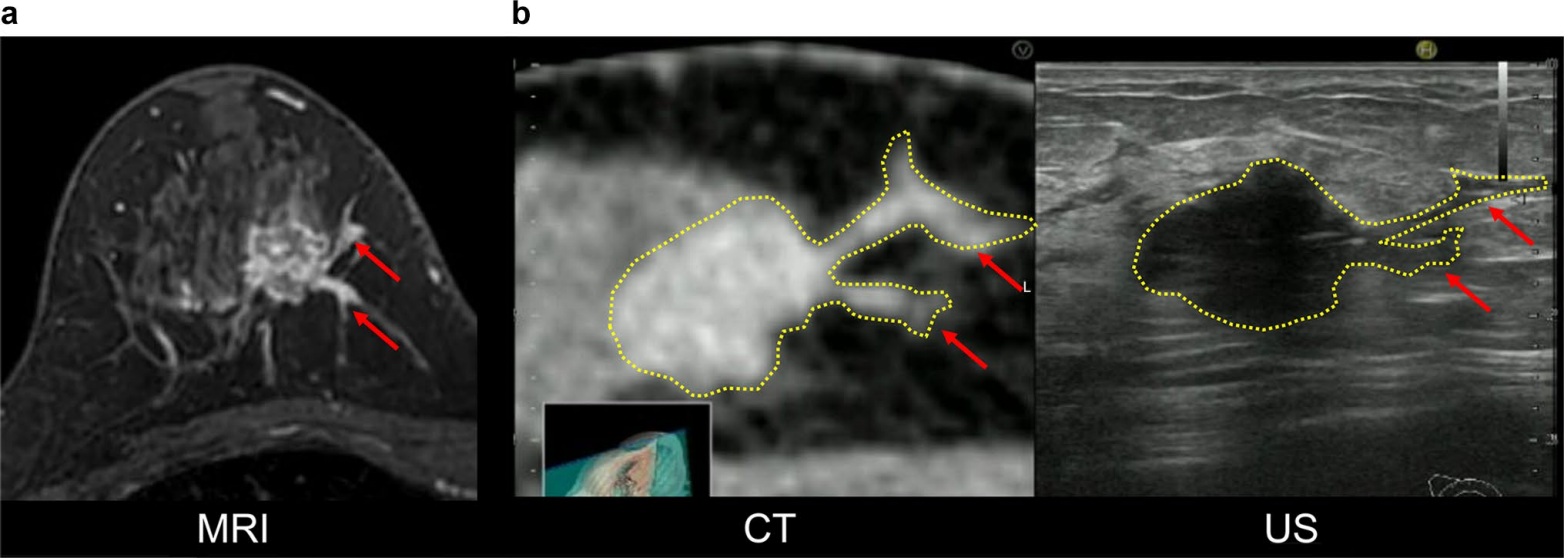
CT/ultrasound fusion image with RVS^®^. a Breast cancer lesions and spread presumed to be an intraductal extension (red arrows) were identified in the prone-enhanced MR image. b After confirming the lesion corresponding to the MR image on the contrast-enhanced CT image, CT and US fusion using RVS^®^ were performed. Fusion imaging made it possible to identify the extent of the spread of the tumor (red arrows) while ensuring objectivity, which identified the extent of the lesion (yellow dotted line) more accurately
